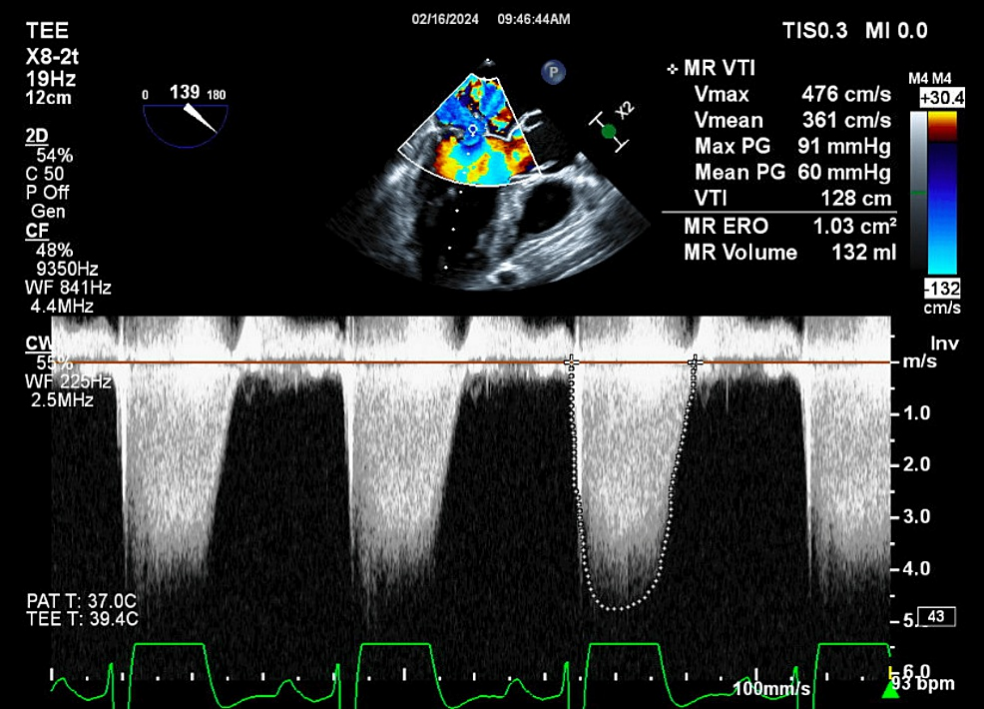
Transesophageal echocardiogram with mitral valve hemodynamics
